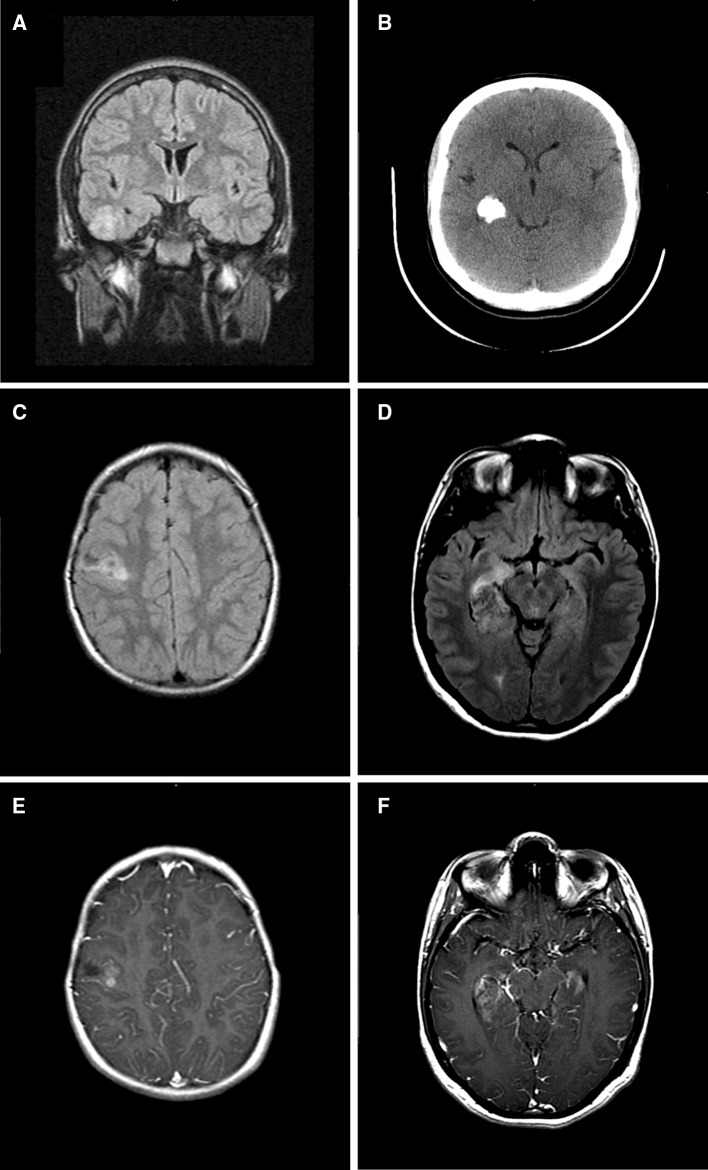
Radiographic features of PLNTY. Typical appearance of a unifocal abnormality best seen on FLAIR sequence (a; case 1). Frequent calcifications appreciated on CT scan (b; case 2). Focal contrast enhancement was seen in two cases (c–f) as demonstrated by FLAIR (c, d) and T1 post-contrast (e, f) images for cases 6 (c, e) and 2 (d, f). Cystic change was also seen in case 6 (c, e)
